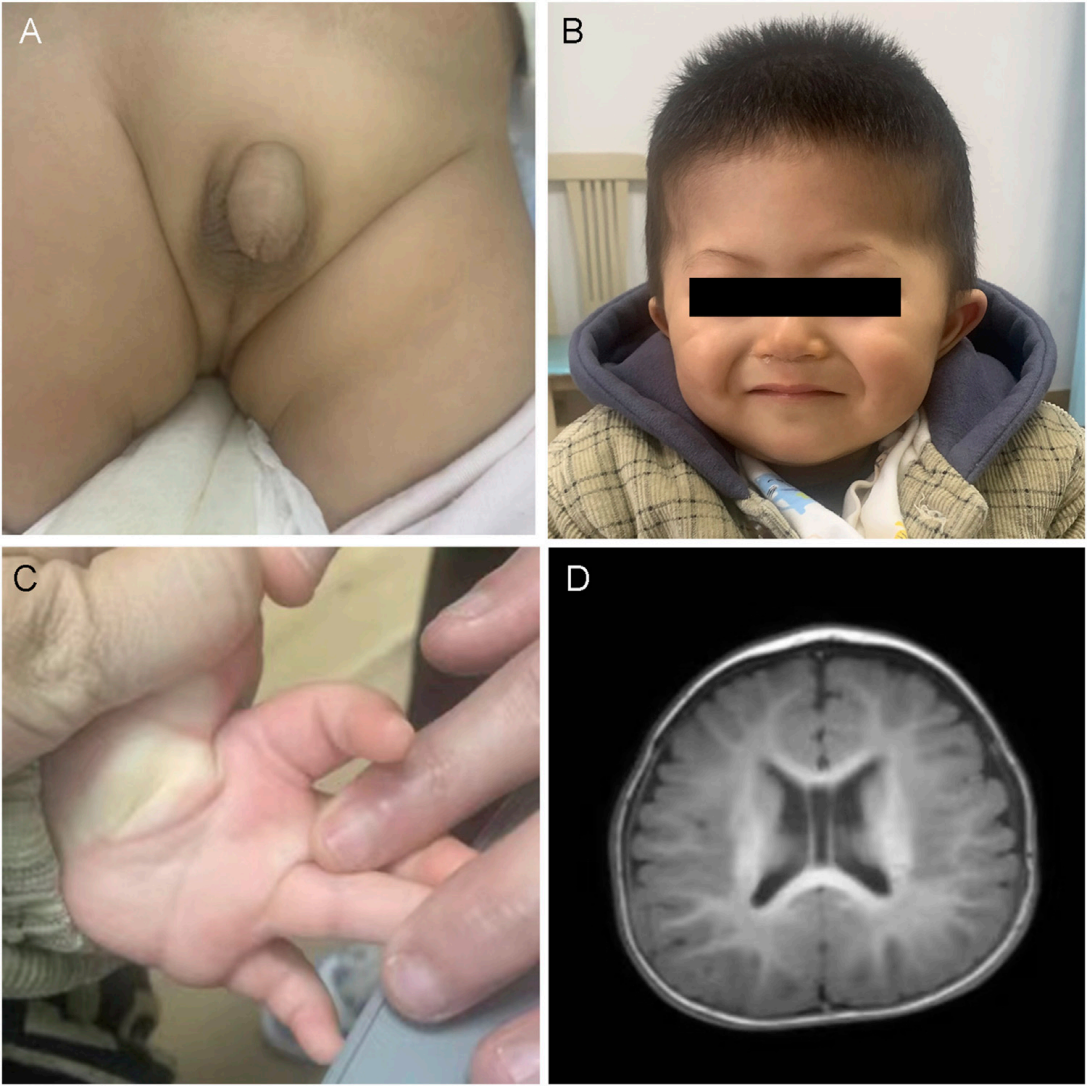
Clinical and imaging features of AII:1. (A) Urinary tract malformation: hypospadias. (B) The photo displays the facial dysmorphic features of AII:1, including a high forehead, synophrys, sparse lateral eyebrows, wide eye spacing, concave eyes, narrow lid fissures, full cheeks, mid-face recession, low nasal bridge, wide and bifid nasal tip, low ear position and thin lips. (C) The transverse palmar crease. (D) Brain MRI demonstrated that the bilateral extracerebral space was widened, the septum pellucidum was wider, and the body of the bilateral ventricles was full.
